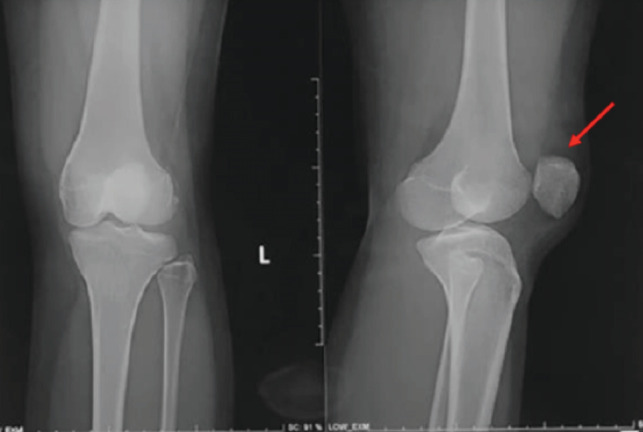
Initial plain radiograph evaluation showing a malposition of the patella with 90° of rotation on the vertical axis as shown by the red arrow.
